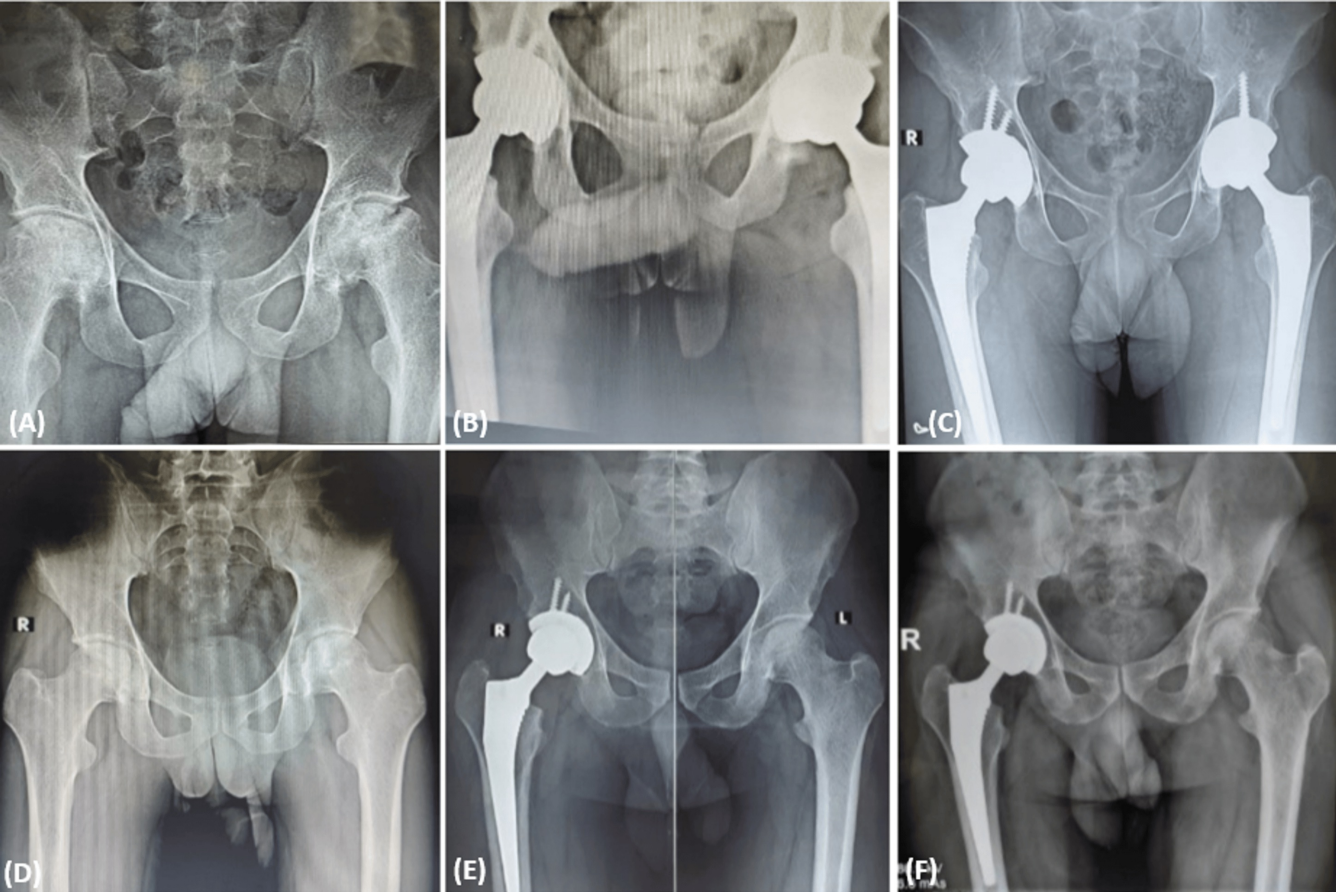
Patient 1- (A) Bilateral ONFH Stage Ⅳ left side and Stage Ⅲ right side; (B) Postoperative radiograph after bilateral uncemented THA; (C) Five-year follow-up showing well-fixed implants in bilateral hips with good functional outcome and no reported complication. Patient 2- (D) Bilateral ONFH Stage Ⅲ right side and Stage ⅡA left side; (E) Managed with uncemented THA for right side and core decompression for left side; (F) Four-year follow-up radiograph showing well-fixed components in the right hip with successful preservation of the native left hip joint with good functional outcome and no reported complication ONFH: Osteonecrosis of femoral head; THA: Total hip arthroplasty
